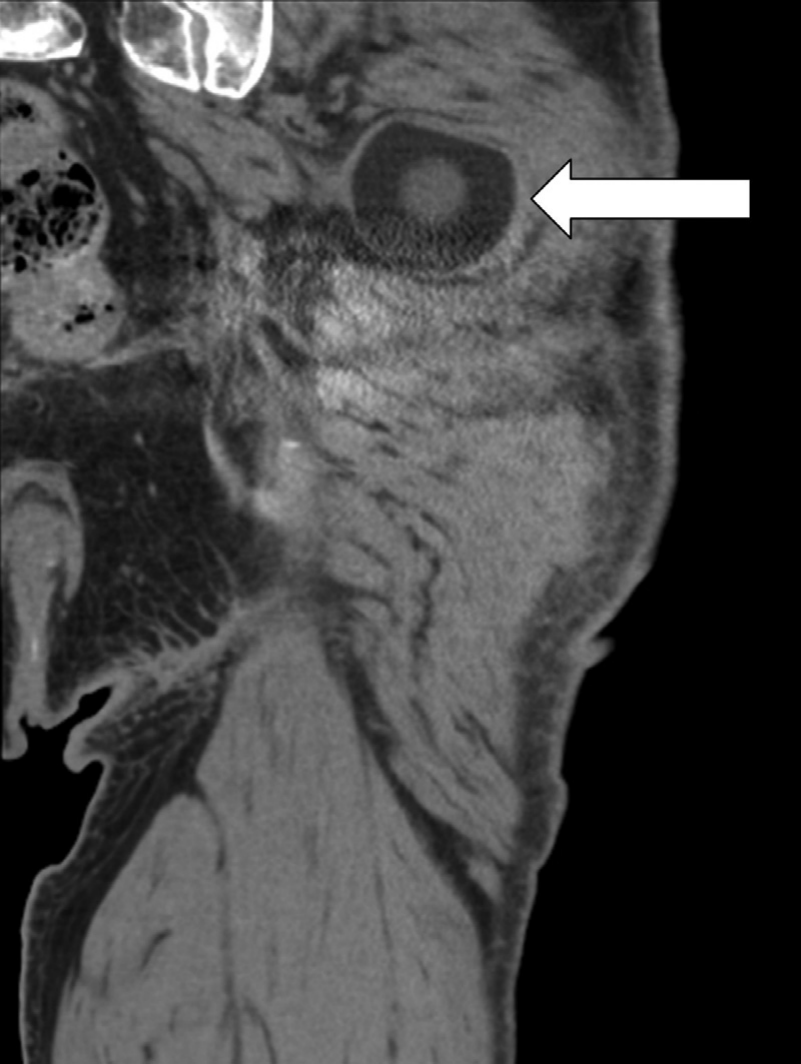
Coronal CT image of the left hip (posterior relative to the previous coronal CT image) shows that the polyethylene head (arrow) is displaced in the posterior gluteal musculature.
